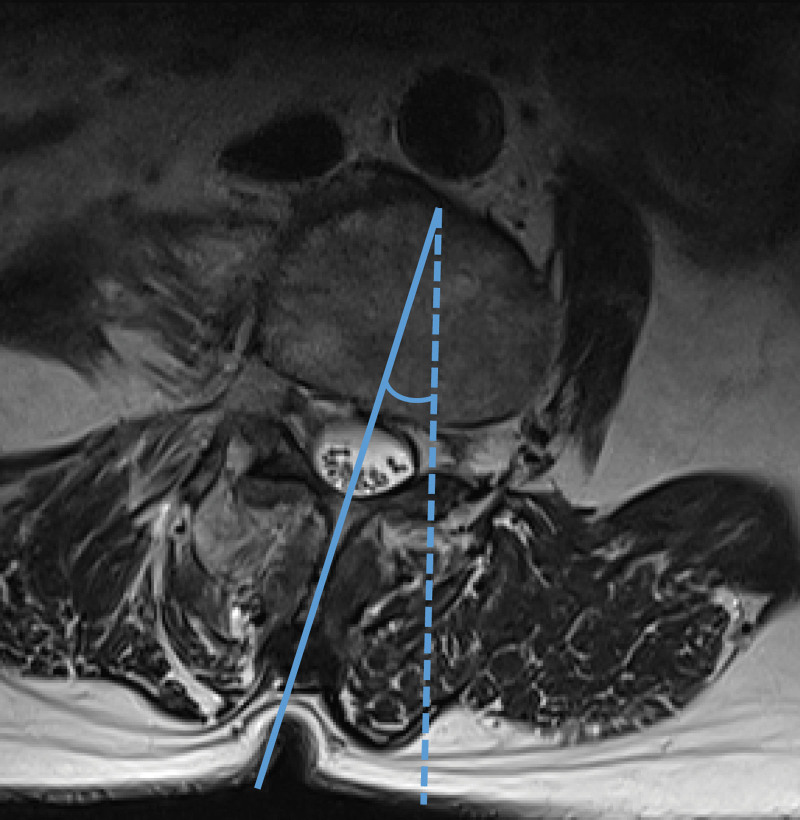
Measurement of VBR in T2 axial image of MRI. VBR was measured the angle formed by 2 lines; the vertical reference line and the lines that passed through the center of the disc and the base of the spinous process in T2 axial image of MRI. VBR = vertebral body rotation, Dotted line indicated the vertical reference line.
